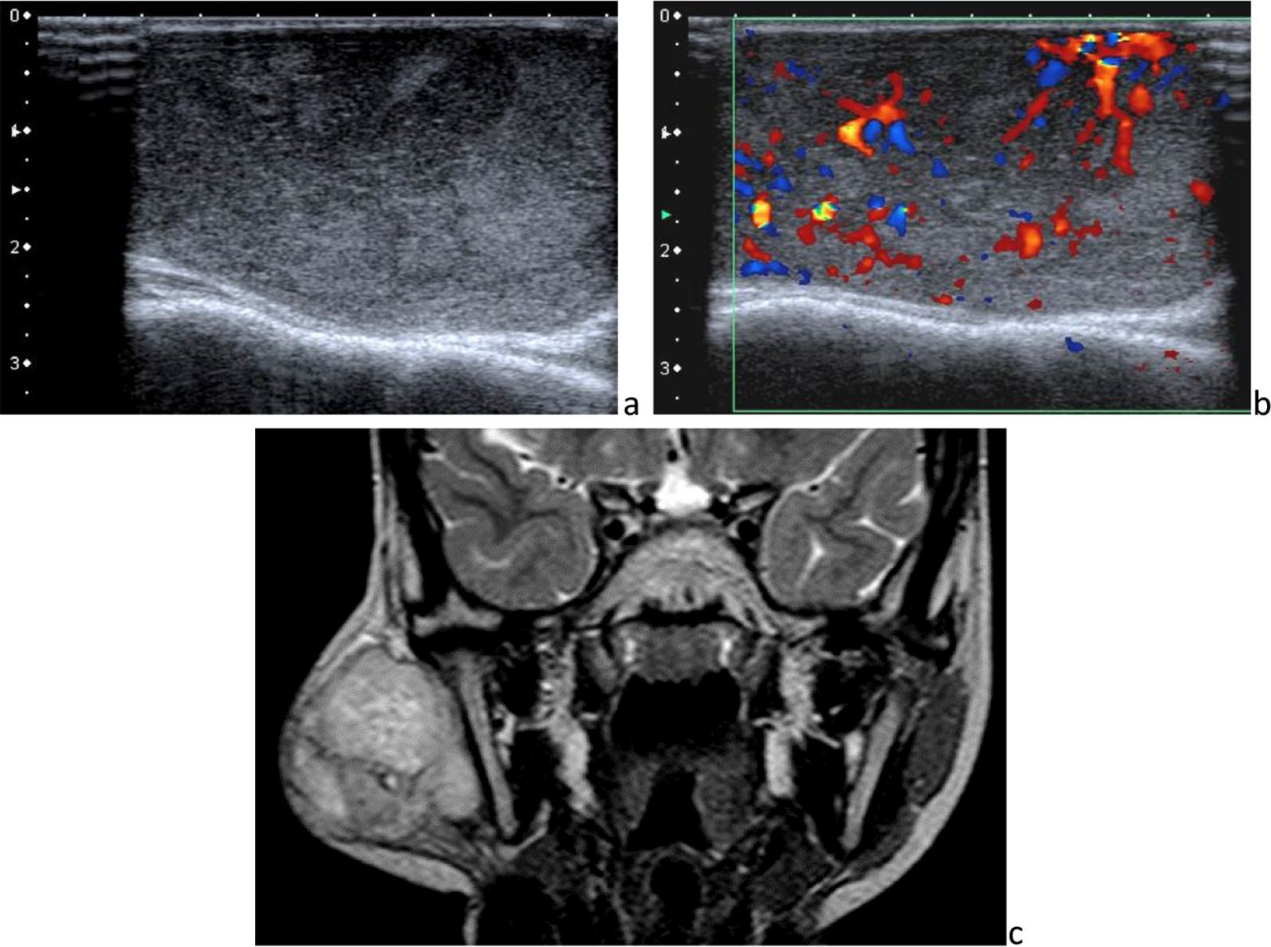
Embryonic rhabdomyosarcoma. Heterogeneous structure and vascularization on B-mode sonography and color Doppler (a and b), large at the time of diagnosis on frontal T_2_-weighted image (c)
